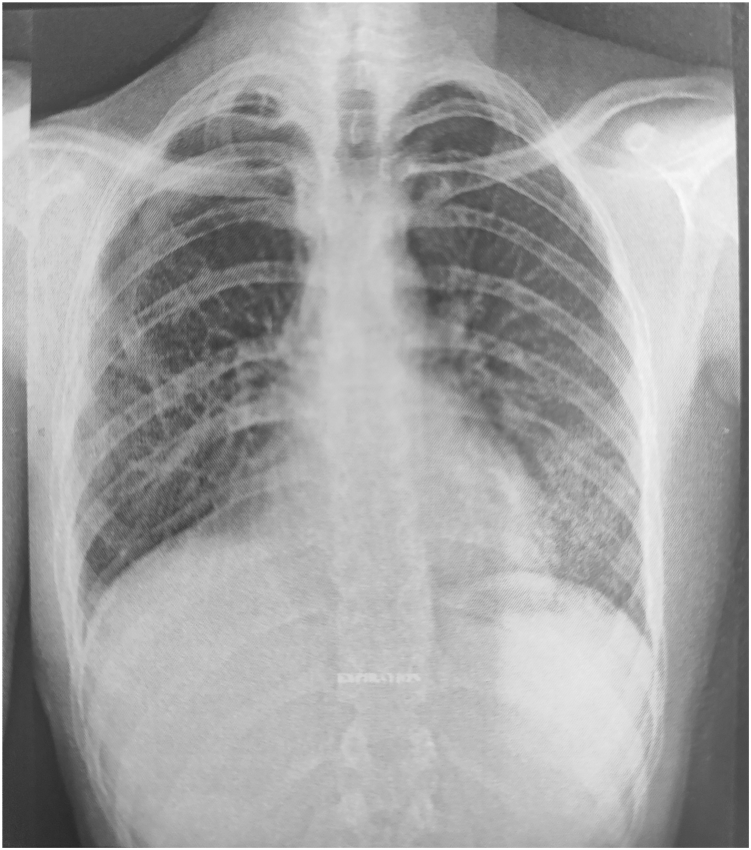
Follow-up chest radiograph at 2 months postoperatively demonstrating complete expansion of the right lung with excellent radiologic outcome.
